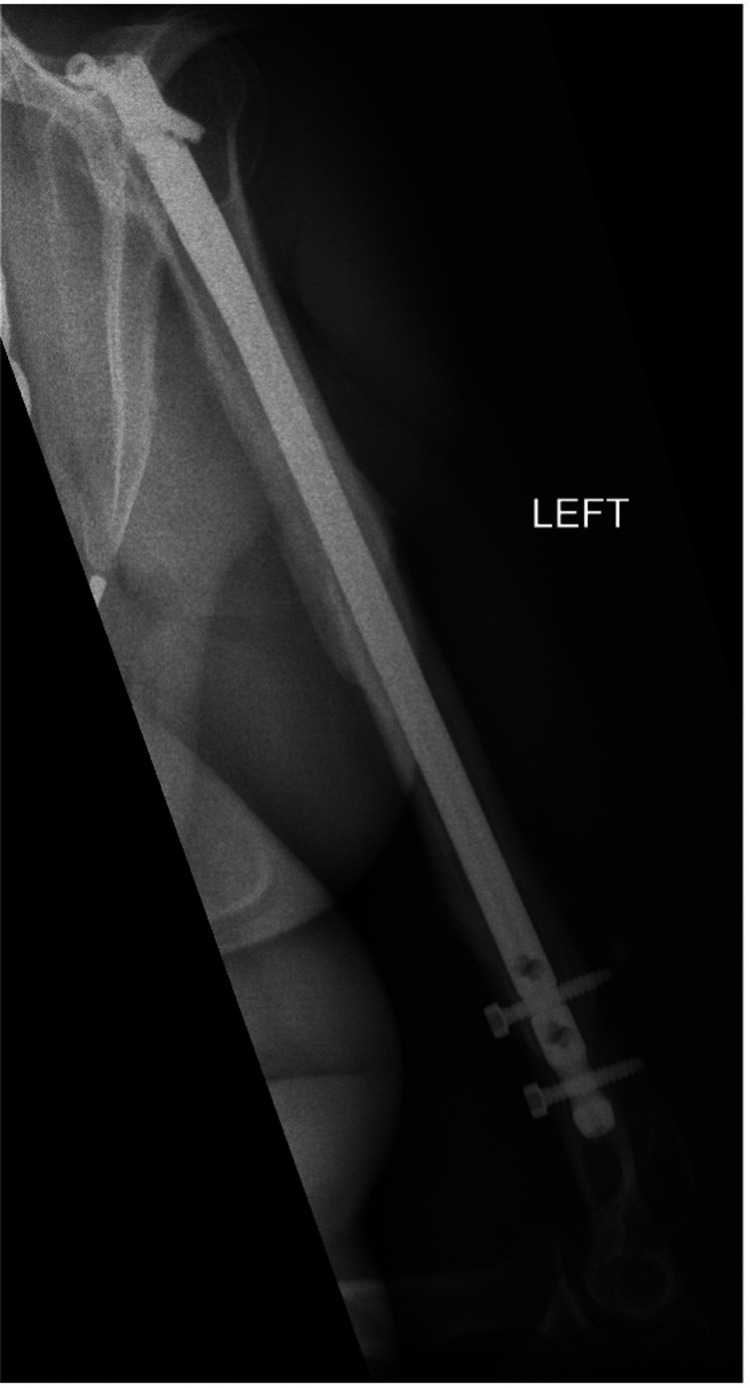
Lateral humerus radiograph demonstrating a healed segmental fracture at two year follow up.
